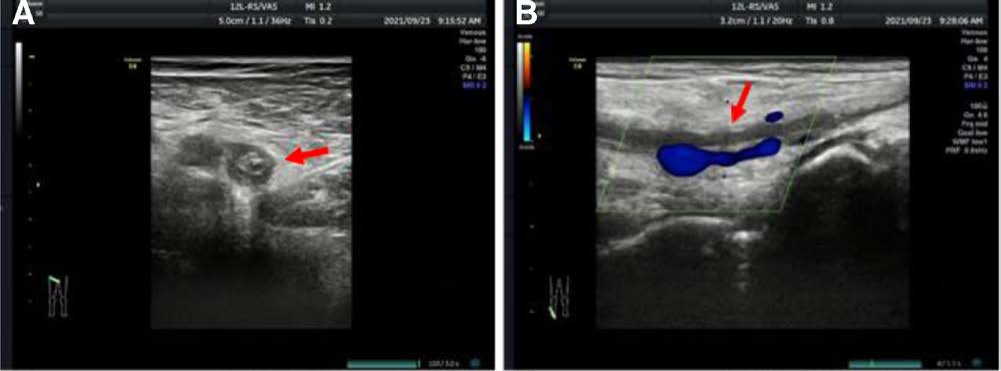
Two-dimensional ultrasonography showed a noncompressible right femoral vein containing hyperechogenicity debris, suggesting right femoral vein thrombosis (A). The right dorsal artery exhibited no blood flow with the color Doppler, indicating right dorsal artery thrombosis (B).
